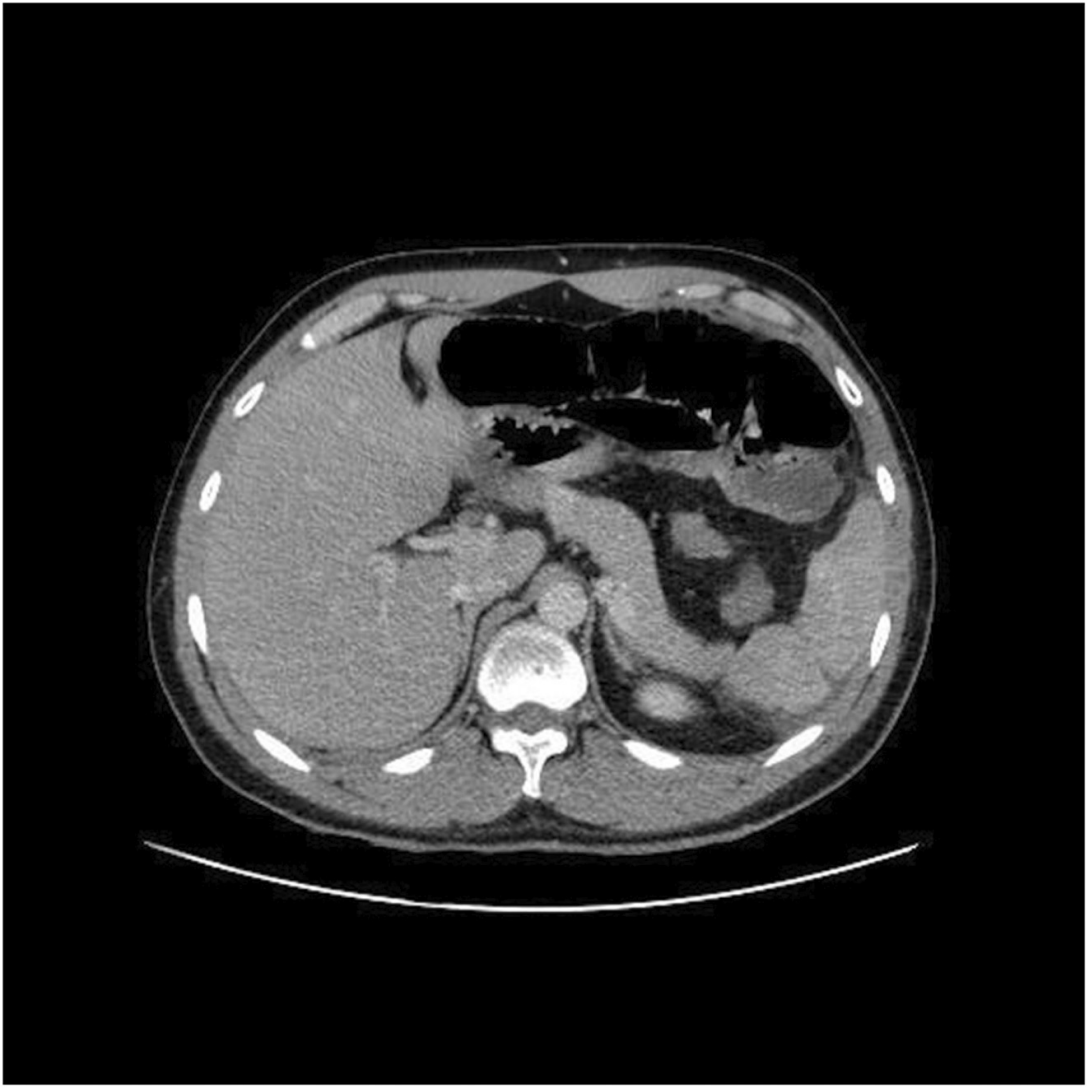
Normal abdominal computed tomography scan with contrast, portal phase, without abnormal pancreatic radiologic findings.
